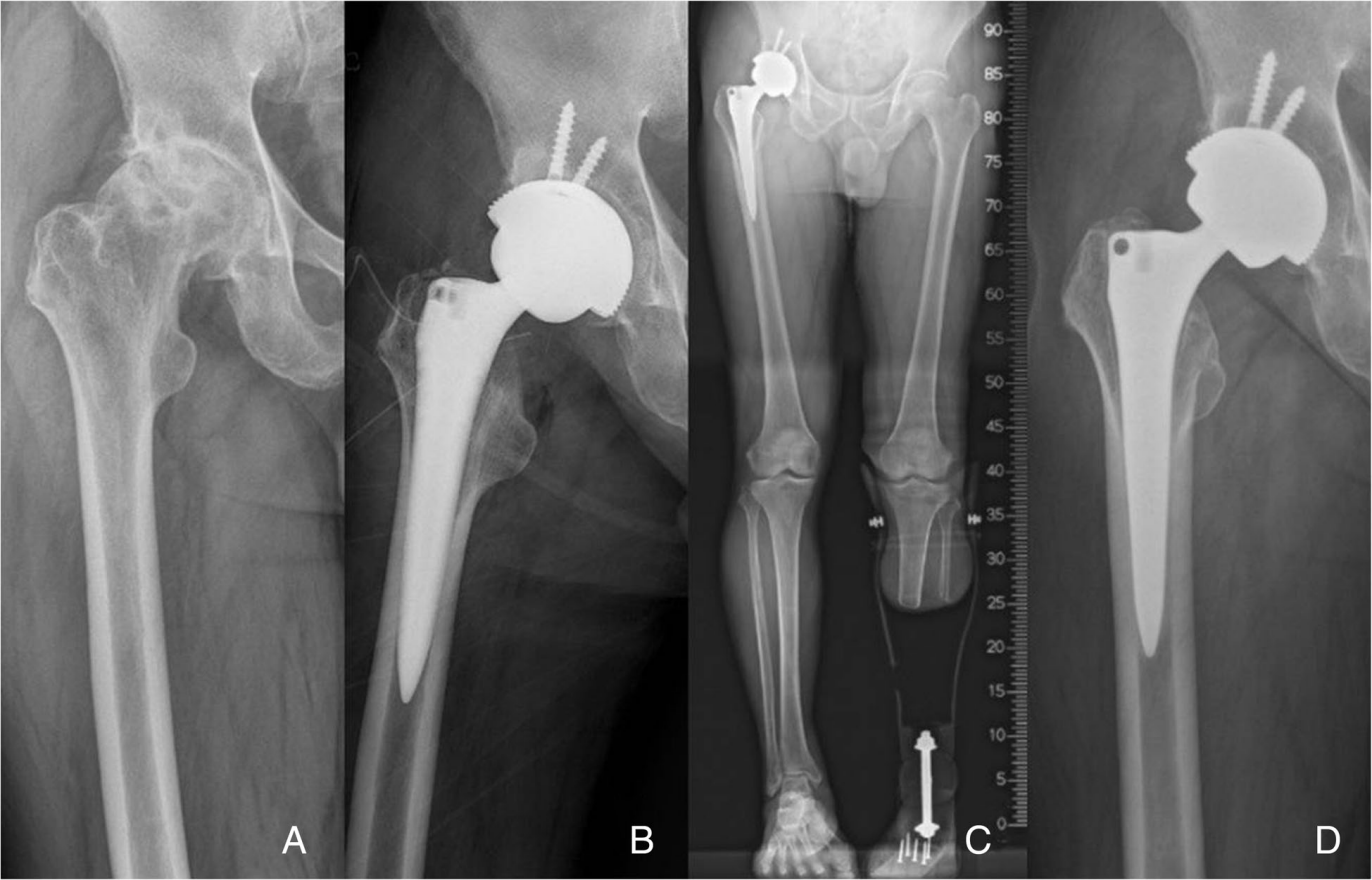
a Preoperative radiograph of a 73-old-year man with primary osteoarthritis of the hip joint. b Postoperative radiograph shows the excellent implant position of cementless total hip arthroplasty using the posterolateral approach. c Postoperative whole lower extremity radiograph of the prosthetic leg taken while standing. d At 5 years after the operation, the radiograph shows stable fixation of the components with a radiolucent line around the proximal femoral stem
